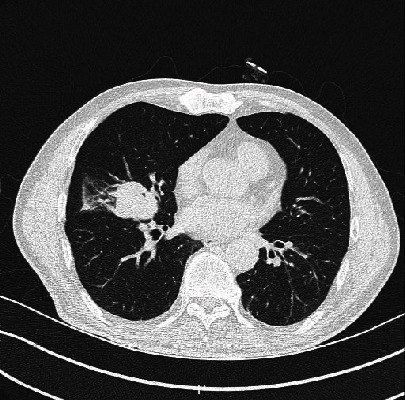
CT imaging of the chest demonstrated a density soft tissue mass in the lateral segment of the right middle lobe.
